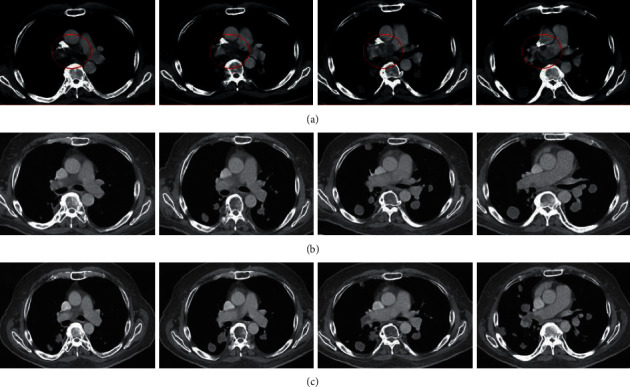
(a) Initial CT of the chest showing large intravascular mass encasing the right main pulmonary artery. (b) Repeat CT chest after 6 cycles of doxorubicin, displaying partial reduction of the primary pulmonary mass and metastatic lesions. (c) After 3 cycles of palbociclib, the patient had stable disease.
